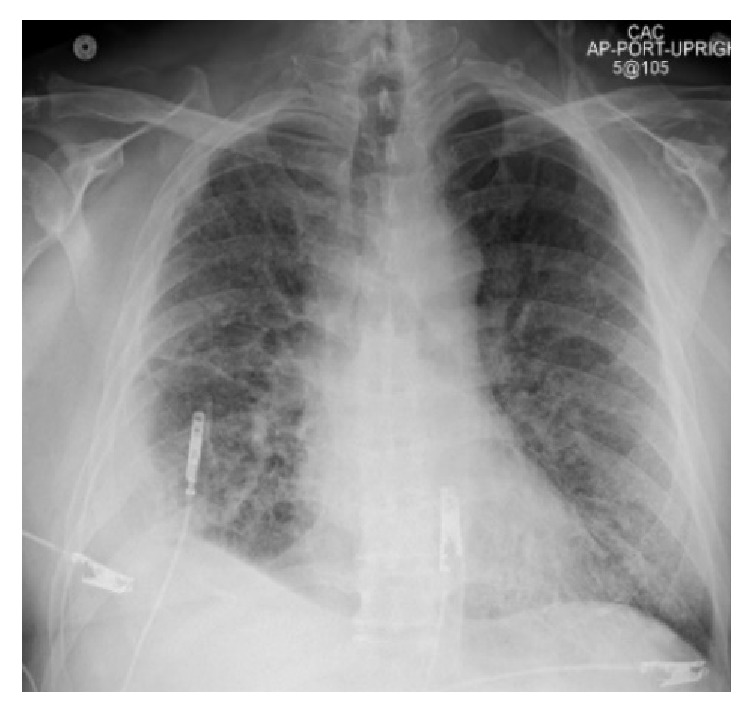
Anteroposterior chest X-ray taken at the hospital admission during acute exacerbation of DIP. Right pleural effusion and bilateral lung interstitial opacities.
